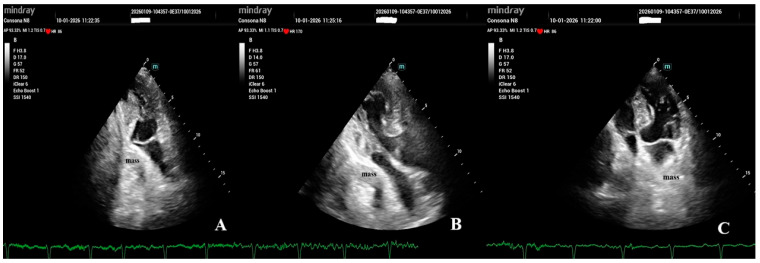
Transthoracic echocardiography showing a hiatal hernia, mimicking cardiac mass. (A) Apical two-chamber view showing a posteriorly located mass. (B) Apical three-chamber view showing the mass in a posterolateral position. (C) Apical four-chamber view showing a mass overlying the left atrium, consistent with a hiatal hernia.
